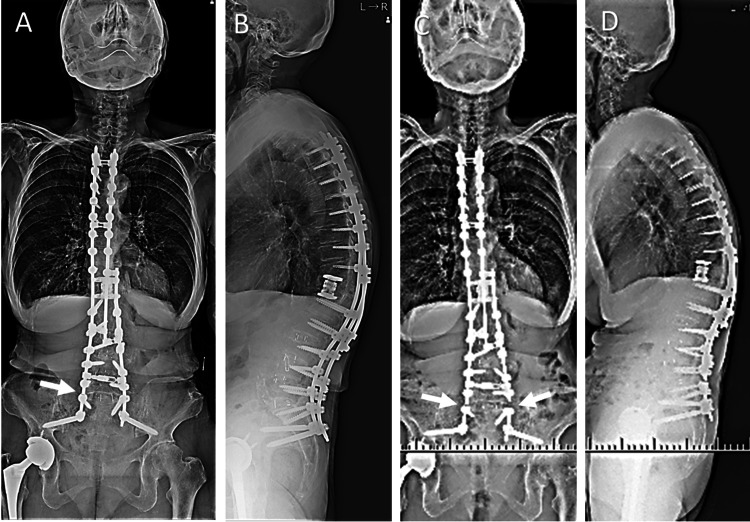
Follow-up images after the second surgery. (A, B) Eighteen months after the second surgery, right-sided RF was incidentally detected on radiographs (arrow) without alignment changes or symptoms. (C, D) Six months after the unilateral RF, bilateral RFs (arrows) occurred with severe left leg pain. RF: rod fracture
